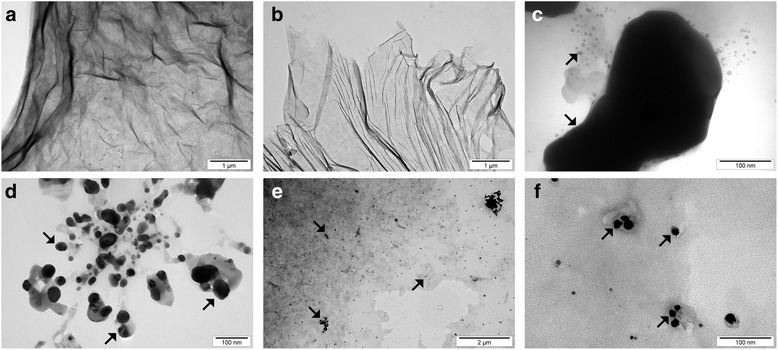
TEM images of agglomerated GO flakes (a), GO flakes after ultrasonic treatment (b), agglomerated Ag-NPs (c), Ag-NPs after ultrasonic treatment (d), and GO-Ag (e, f). The decrease of the average GO particle size after ultrasonic treatment was caused by defragmentation or folding of the GO flakes. The decrease of the average Ag size after ultrasonic treatment was caused by defragmentation of Ag agglomerates. Note: Arrows point to Ag-NPs/agglomerates
